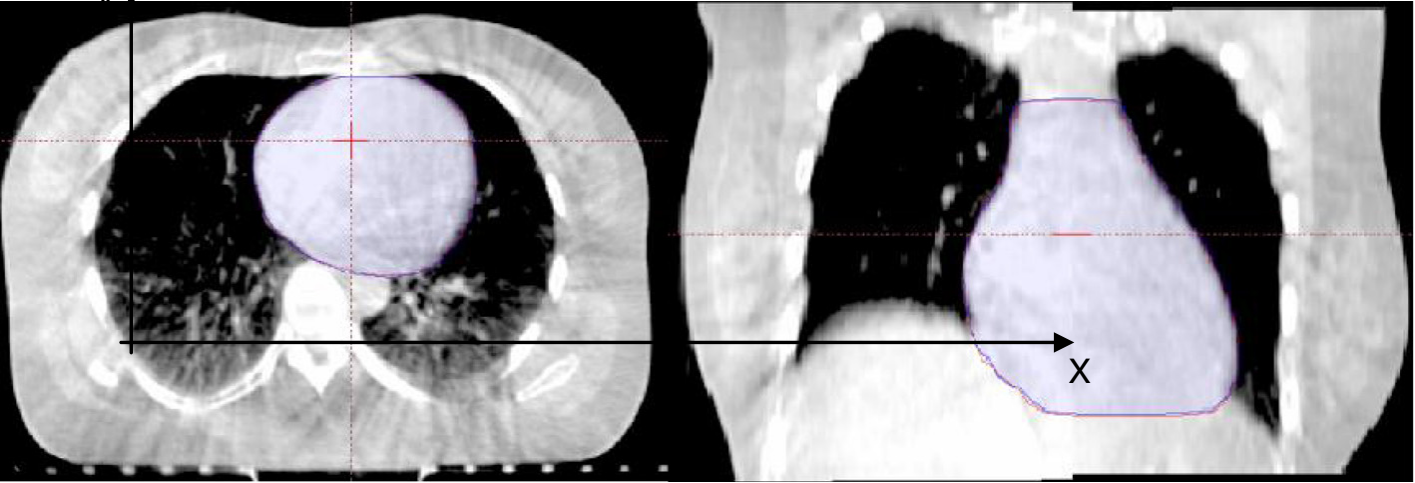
Reproducibility of CBCT images. CBCT images of the heart from one representative patient show overlap of the anatomical region. The reproducibility of the heart between the first CBCT (red line) and other images (blue line) are shown on the sectional and coronal planes.
